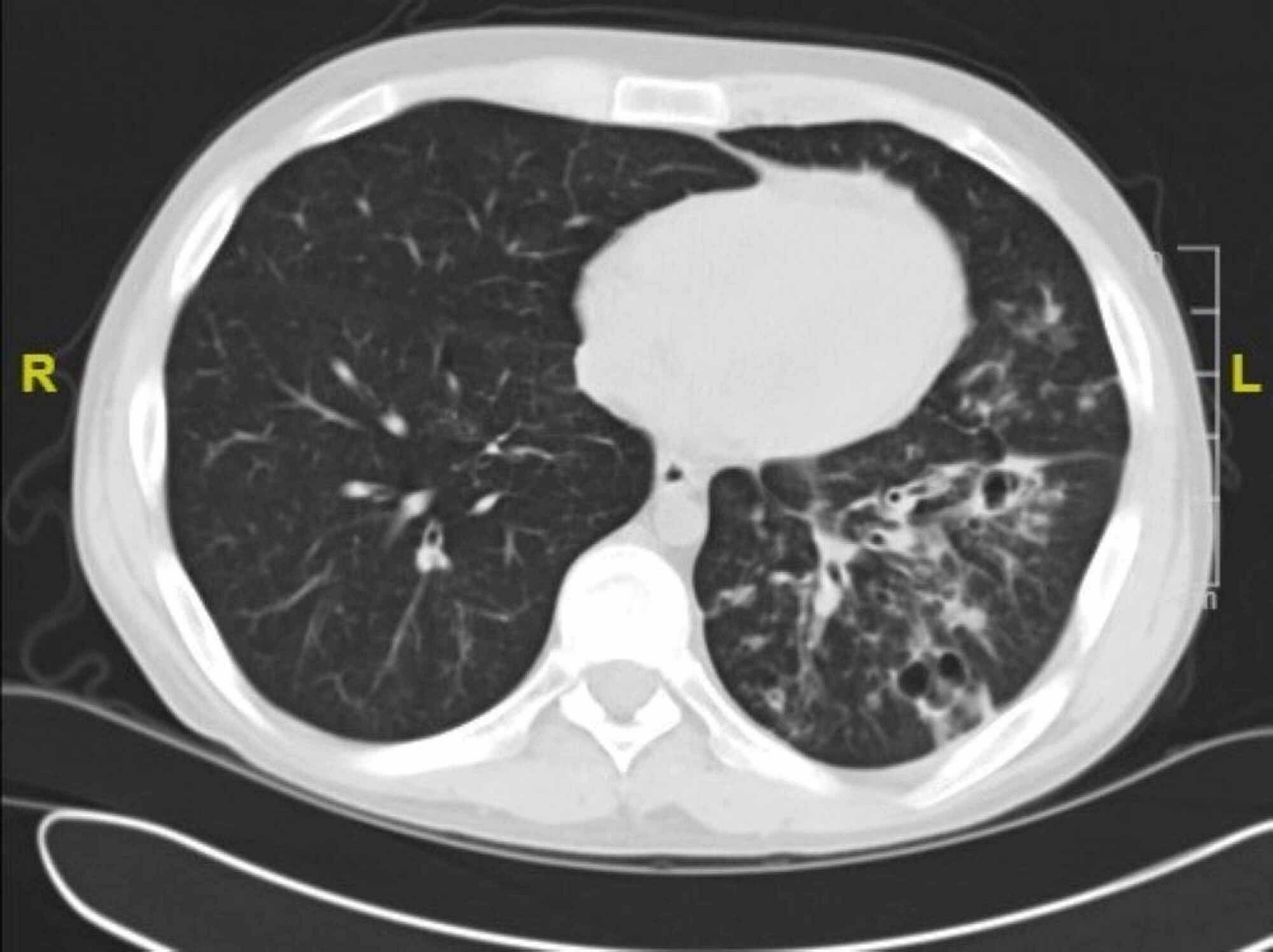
CT chest CT chest demonstrating persistence of cavitary lesions due to prior tissue necrosis.
